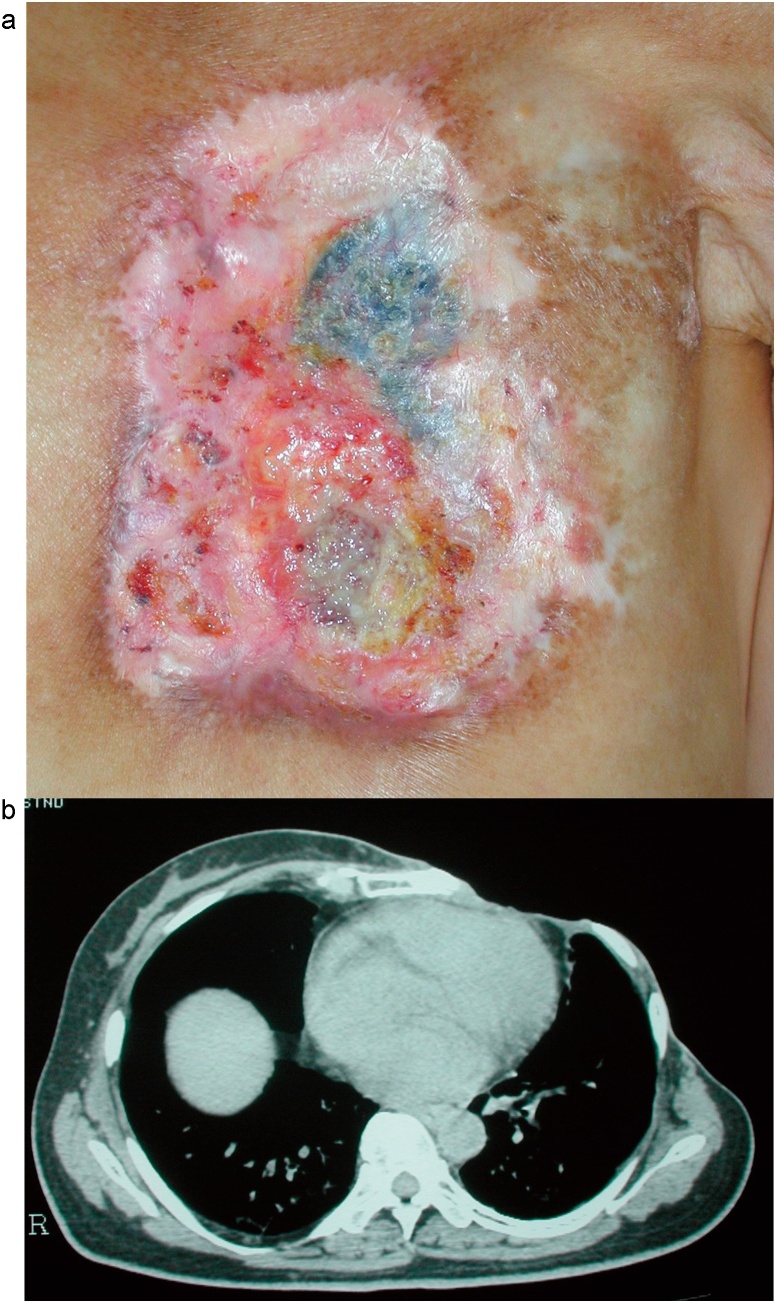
(a) Findings obtained when the patient visited our hospital. The ulcer affecting the left chest wall extended from the subclavian to xiphoid levels. The third, fourth, fifth, and sixth costal cartilages and bones were missing, and parts of the lung and pericardium could be seen. (b) Computed tomography image. The ulcer was deep enough to expose the pericardium. Computer tomography image. There is only a small gap between the ulcer and the heart.
